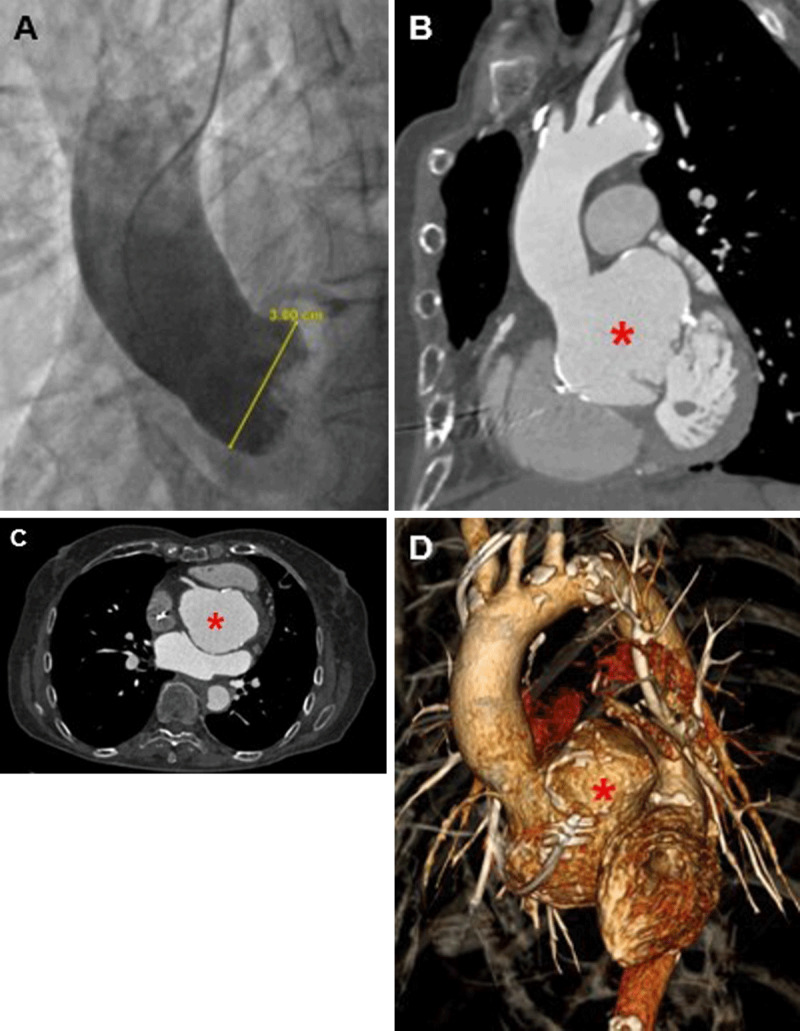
(A) Preoperative invasive angiography demonstrates an aortic root of normal diameter. (B, C) Computed tomography (CT) and (D) 3-dimensional reconstruction of CT scan performed 9 years after bioprosthetic aortic valve replacement shows a giant isolated aortic root aneurysm (red asterisk).
